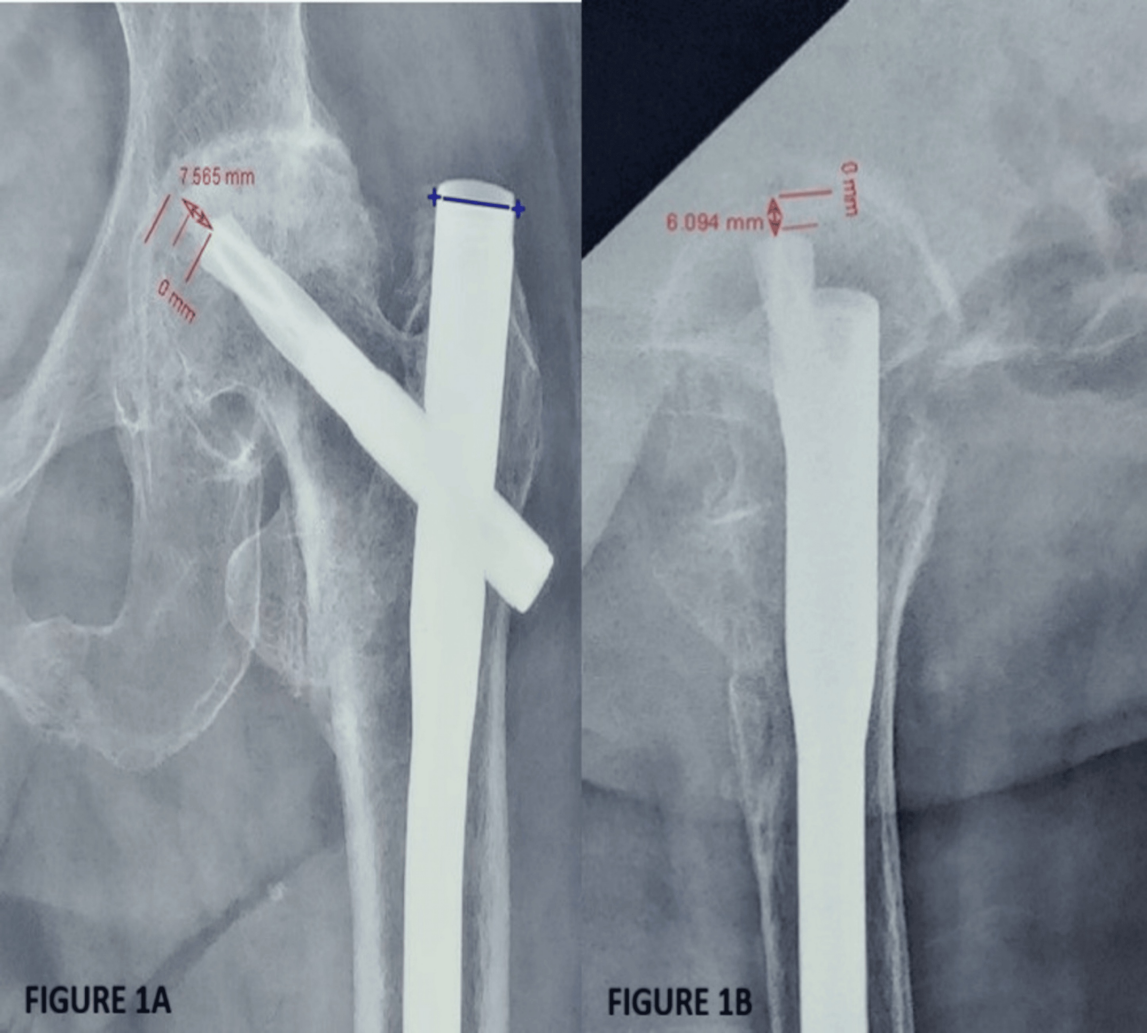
Tip-apex distance calculation on anteroposterior and lateral radiographs. (A) Anteroposterior view. (B) Lateral view. The tip-apex distance is calculated as the sum of measurements in anteroposterior and lateral radiographs. Blue line = reference measurement (proximal nail diameter = 16.5 mm).
